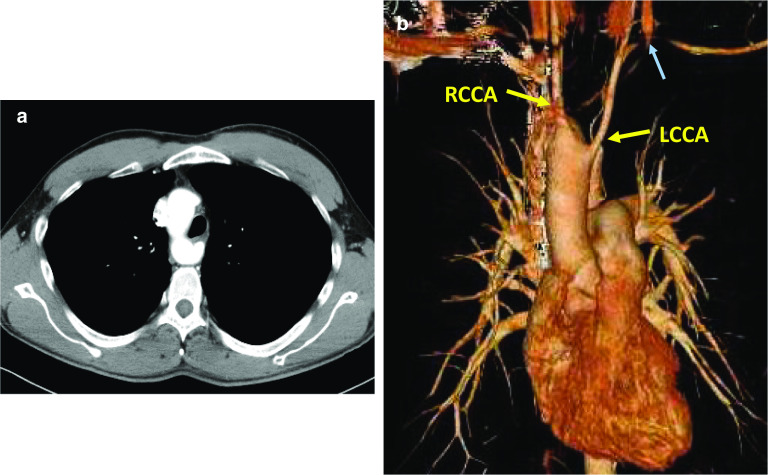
Three-dimensional CT revealed an RAA with stenosis of the left subclavian artery. Contrast-enhanced CT revealed right aortic arch (a). The left common carotid artery can be seen branching off the aortic arch prior to the right common carotid artery (b). A stenosed left subclavian artery was detected (blue arrow), and this case was classified as Edwards IIIC
